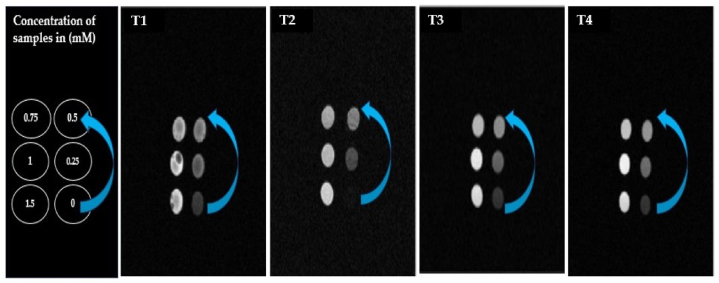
(T1, T2, T3 and T4). Shows T1 bright contrast MR images of MSF-NPS at (0, 0.25, 0.5, 0.75, 1 and 1.5 Mm).
